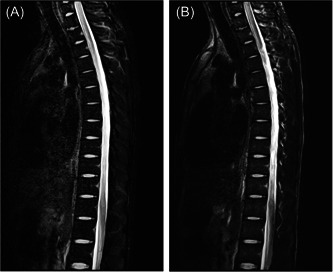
Both (A) and (B) are magnetic resonance imaging of acute transverse myelitis
